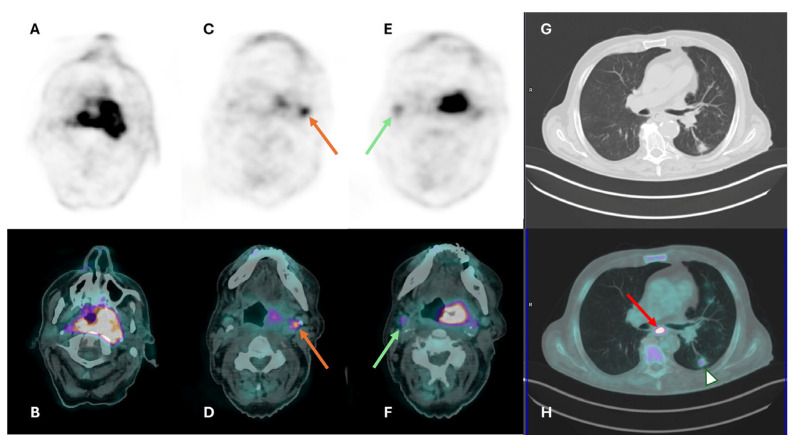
Axial ^18^F-FDG PET (A,C,E), CT (G), and fused PET/CT images (B,D,F) for staging in a patient with oropharyngeal carcinoma. Increased ^18^F-FDG uptake is seen in the primary tumor (A,B) with bilateral pharyngeal involvement (>on the left), as well as in bilateral cervical lymph nodes (yellow and green arrows in (C,D,E,F)). The focal ^18^F-FDG uptake in the esophagus (red arrow in (H)) was confirmed as a synchronous primary. Slight uptake by a pseudo-nodular left lung consolidation (white triangle in (H)) was due to inflammatory changes.
